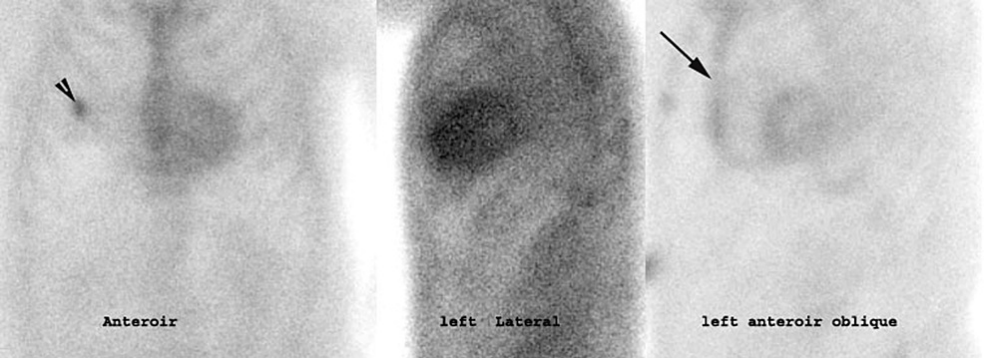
Anterior, left lateral, and left lateral oblique planar images of technetium-99m pyrophosphate (Tc-99m PYP) scintigraphy Anterior, left lateral, and left lateral oblique planar images of technetium-99m pyrophosphate (Tc-99m PYP) scintigraphy showing diffuse sternal uptake (arrow) and focal uptake in the right rib cage consistent with previous right rib fracture (arrowhead); in the left anterior oblique view, the appearance of the sternal uptake mimics uptake in the right ventricle
